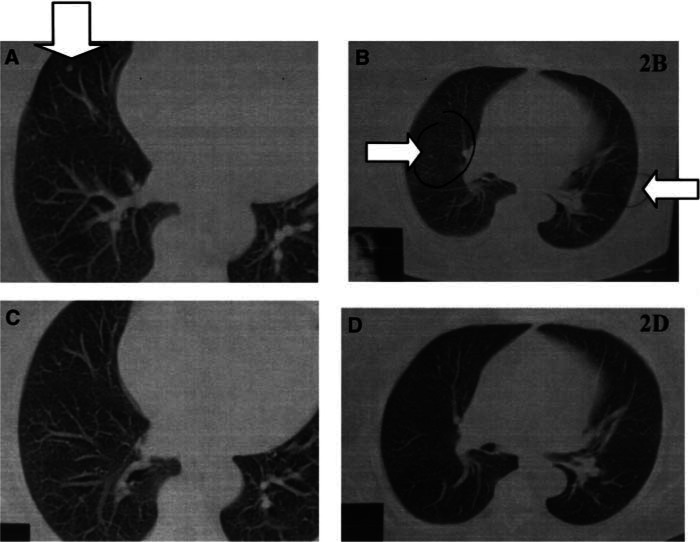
Patient 9: CT of the thorax. (A and B). Three metastatic deposits <1 cm. (C and D). At 3 months after initiation of autologous melanoma vaccine with low dose and inhalations of IL-2–significant partial regression of all lesions. Patient has eventually achieved CR.
